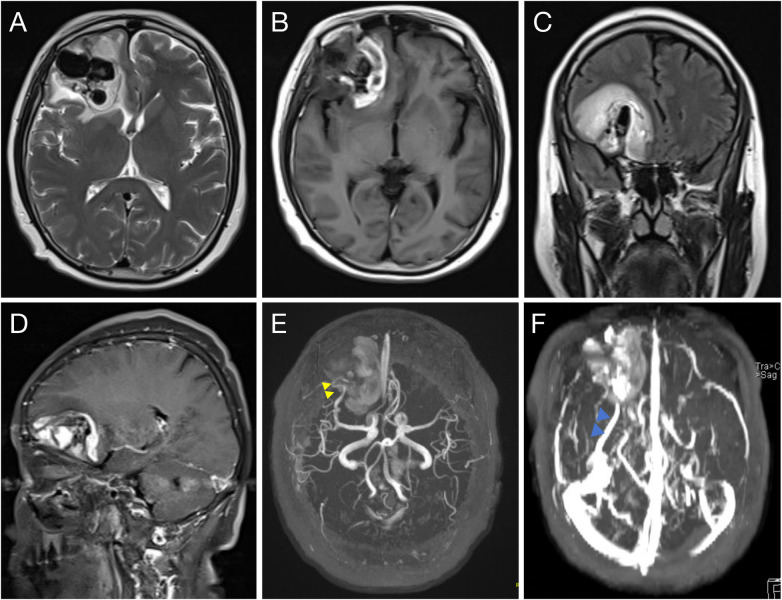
Preoperative T2-weighted MRI shows multiple prominent flow voids in the right frontal region (A). T1-weighted MRI demonstrates right frontal intraparenchymal hemorrhage with heterogeneous signal within the hematoma (B) FLAIR shows marked perilesional edema (C). Contrast-enhanced T1 MRI reveals heterogeneous enhancement (D). MRA identifies an arterial branch from the right MCA M2 segment coursing through the lesion (yellow triangle) (E) MRV shows an enlarged draining vein (blue triangle) (F).
